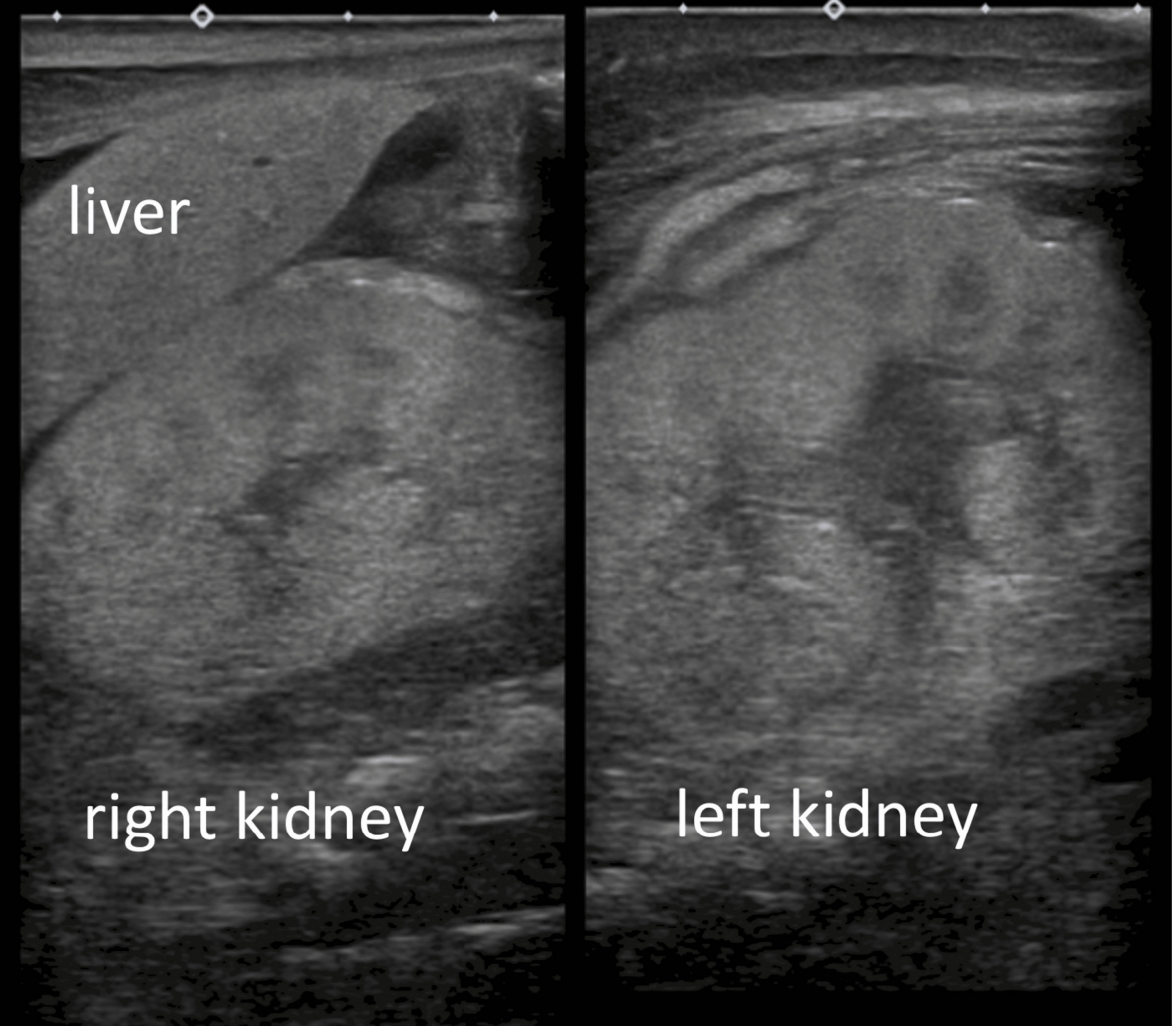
The ultrasound image of the kidney on admission. Both kidneys were highly echoic, with reduced corticomedullary differentiation.
